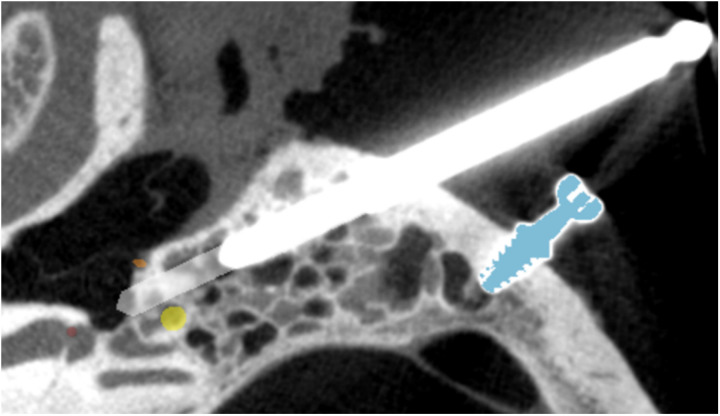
Intra-operative cone-beam CT of titanium rod placed inside the drilling trajectory and calculation of the facial nerve (yellow) distance using a dedicated 3D planning software programme. Fiducials (blue) are used for patient to image registration. The chorda tympani (orange) and the target/cochlea (red) are also visualized.
